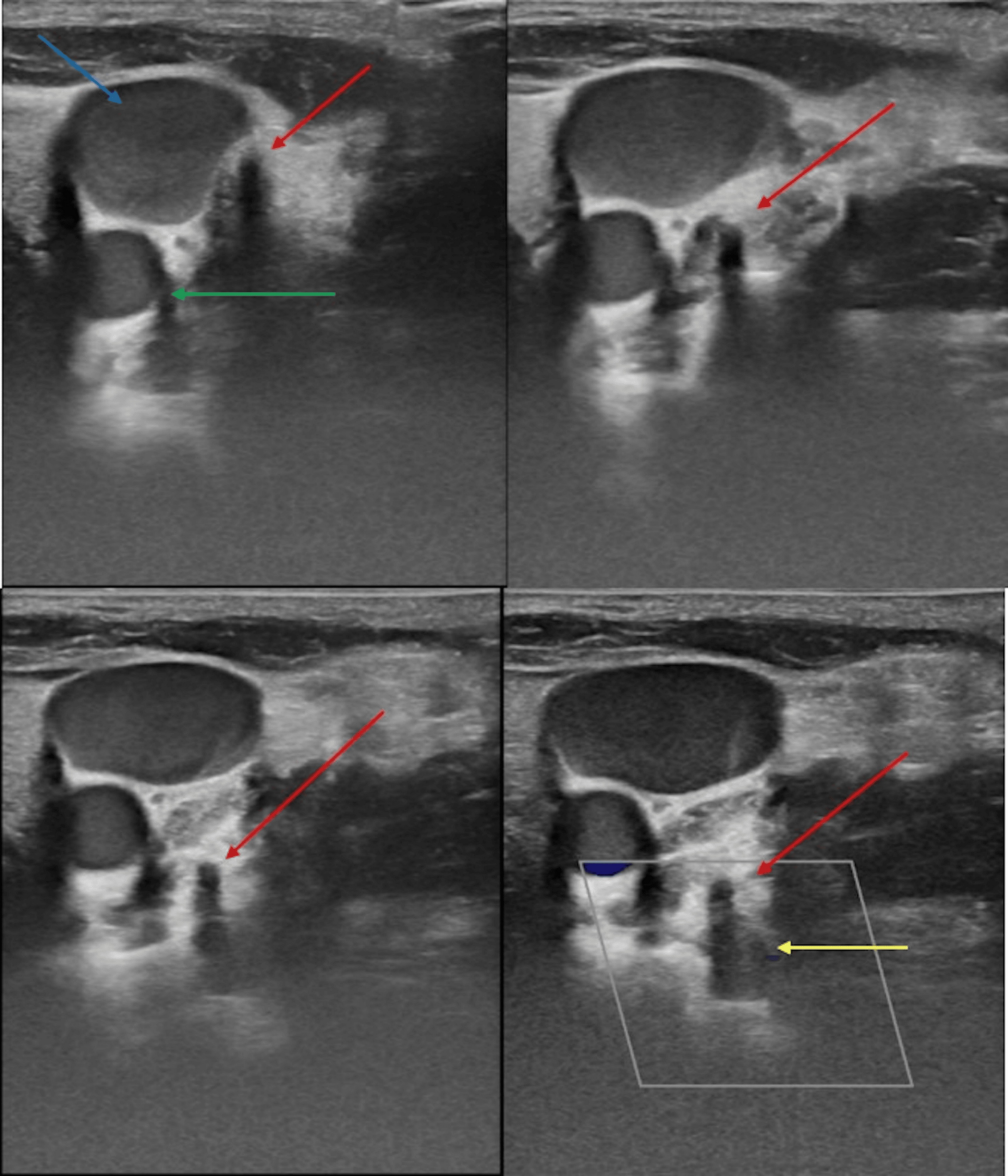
Serial ultrasound images depicting the central venous catheter (red arrow) showing posterior acoustic shadowing, coursing just posterior to the internal jugular vein (blue arrow) down to the vertebral vein and superficial to the vertebral artery (yellow arrow). The common carotid artery is also seen (green arrow).
